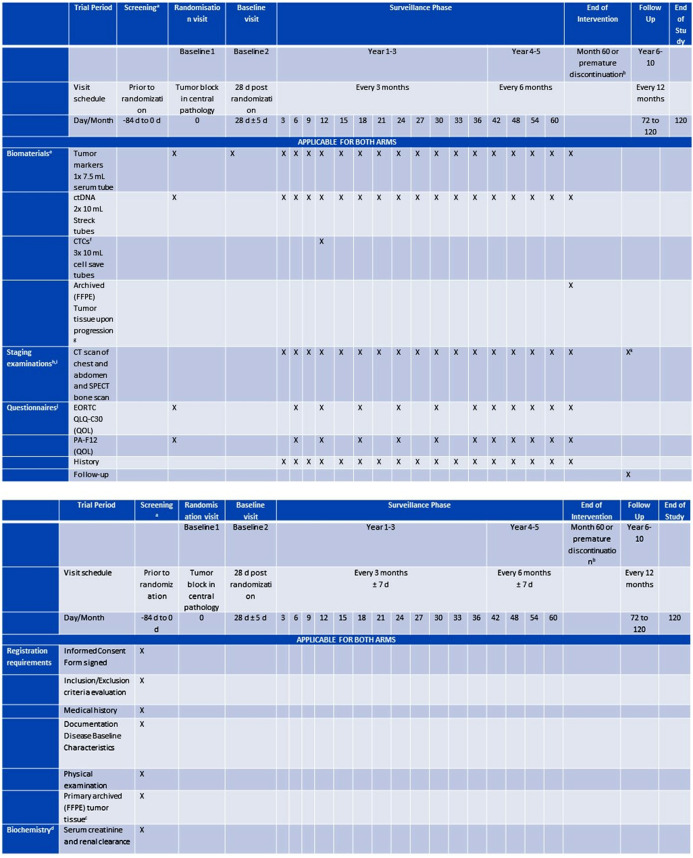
SPIRIT schedule of enrollment, interventions, and assessments. a: Results of screening tests or examinations performed as standard of care prior to obtaining informed consent but within the timelines may be used rather than repeating required test; b: Premature study intervention discontinuation due to either of the following: 1. via imaging verified recurrence, 2. Pregnancy, 3. safety or medical reasons, 4. patients’ withdrawal of consent; c: Primary tumor tissue according to the requirements stated in the study protocol section 11.2. Important note: in case of bilateral breast cancer, tissue from both sides is needed. For multifocal breast cancer: tissue sample of one focus is sufficient, if the tumor biology among the foci does not differ. However, if the tumor biology among the foci differs, tissue of the therapy-defining focus, according to the investigator, should be used; d:Results of serum creatinine and renal clearance performed within 84 days prior to obtaining informed consent may be used rather than repeating required test; e: Materials for blood collection and transportation devices will be provided by the Central Study Center at Dep. Ob/Gyn University Hospital Ulm; f: Mandatory at Month 12, additionally required at visit after first verified tumor marker increase, whenever that occurs; g: Highly endorsed in case of detection of recurrence/metastatic disease; h: Referral to radiologist from investigator or aftercare specialist (gynecologist/family physician/oncologist) – Can be triggered by either of the following: 1. clinical indication (both arms), 2. increase in tumor markers (only intensive surveillance arm), 3. if presence of ctDNA was detected (only intensive surveillance arm); i: If CT and SPECT not possible due to, e.g., allergy to the contrast agent: PET scan or whole-body MRI, Complementary imaging tests if indicated; j: Available on paper, handed out at study visits; k: Staging examinations or Breast Imaging obtained during the standard aftercare visits should be documented in the eCRF (e.g., Bilateral mammography and supplementary bilateral breast ultrasound with axillary lymph nodes (where applicable) and/or MRI of the breasts.
